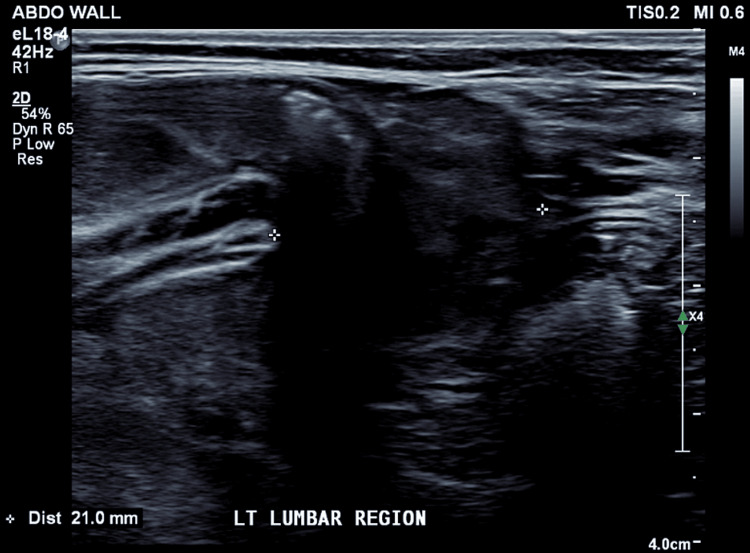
Ultrasound image of the left lumbar region, which demonstrates the hernia containing bowel.
